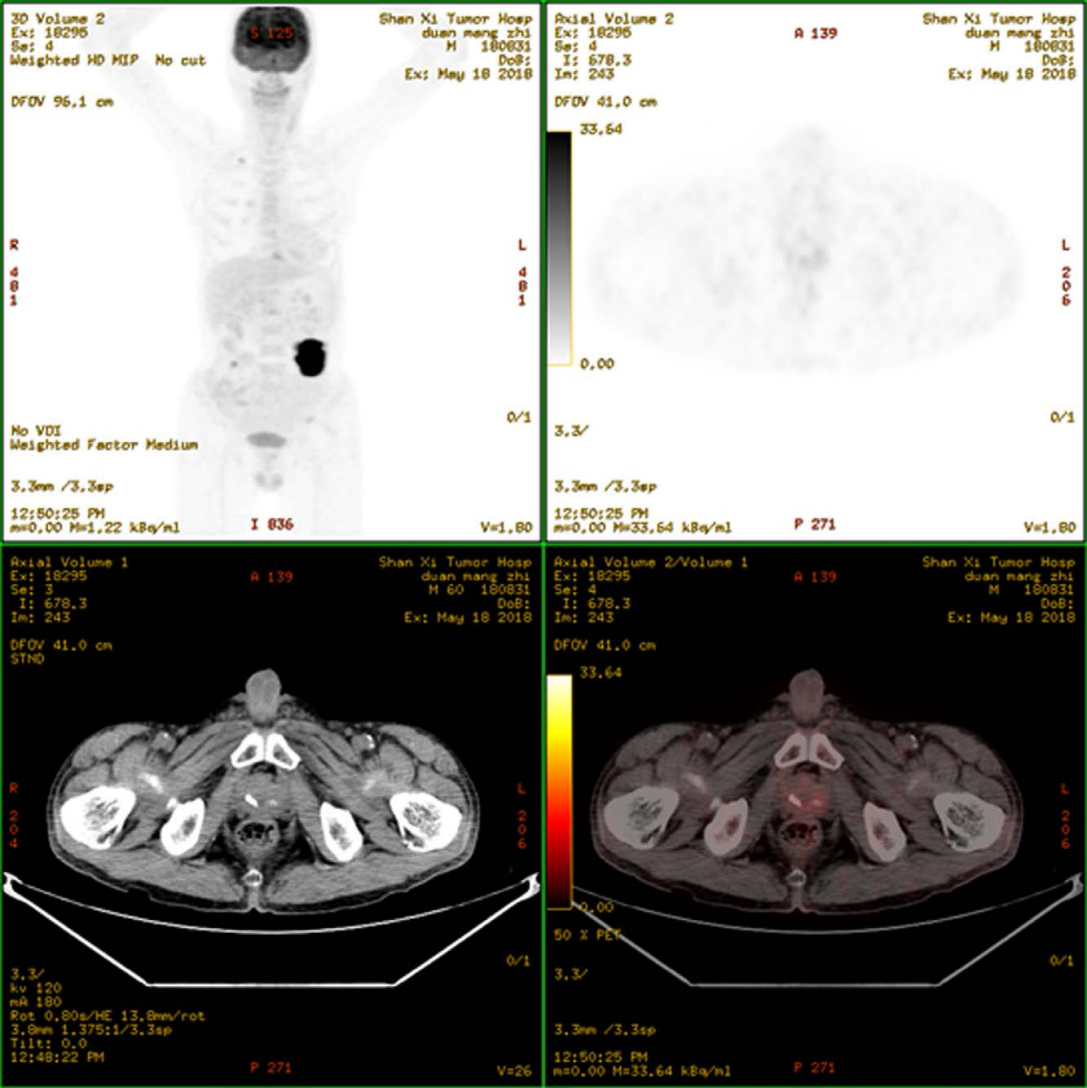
^18^F-FDG PET/CT scan before chemotherapy. ^18^F-FDG PET/CT = ^18^F-fluorodeoxyglucose positron emission tomography/computed tomography.
